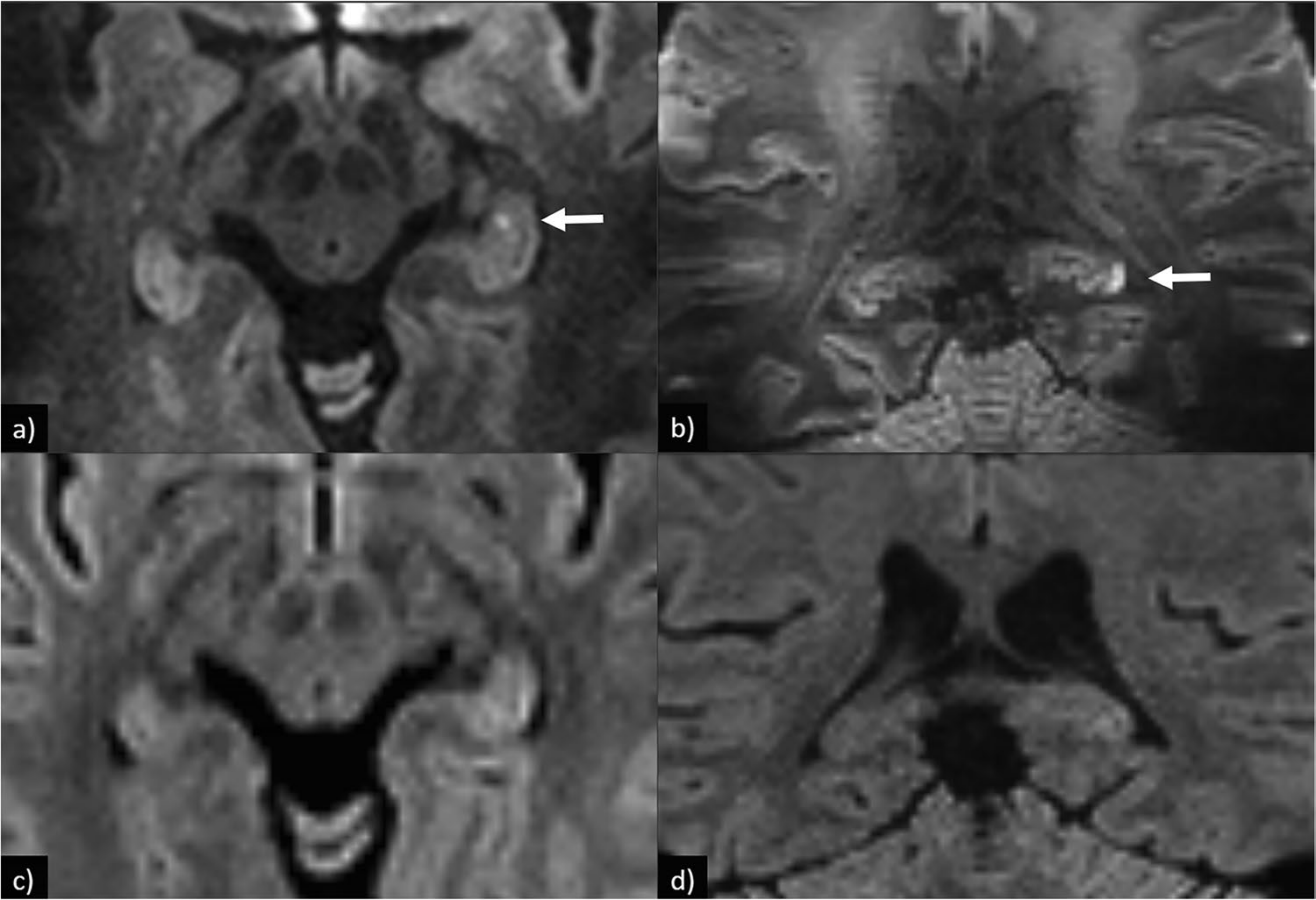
DWI for patient no. 4 with two left-sided hippocampal DWI-lesions at 7 T but not 3 T. All DWI are RESOLVE. a 7 T, DWI, transversal 2 mm, slice gap 0.6 mm, pixel size 0.50 × 0.50 mm^2^; b 7 T, DWI, coronal 2 mm, slice gap 0.2 mm, pixel size 1.02 × 1.02 mm^2^; c 3 T, DWI, transversal 3 mm, slice gap 0.6 mm, pixel size 1.33 × 1.33 mm^2^; d 3 T, DWI, coronal 2 mm, slice gap 0.2 mm, pixel size 1.15 × 1.15 mm^2^
